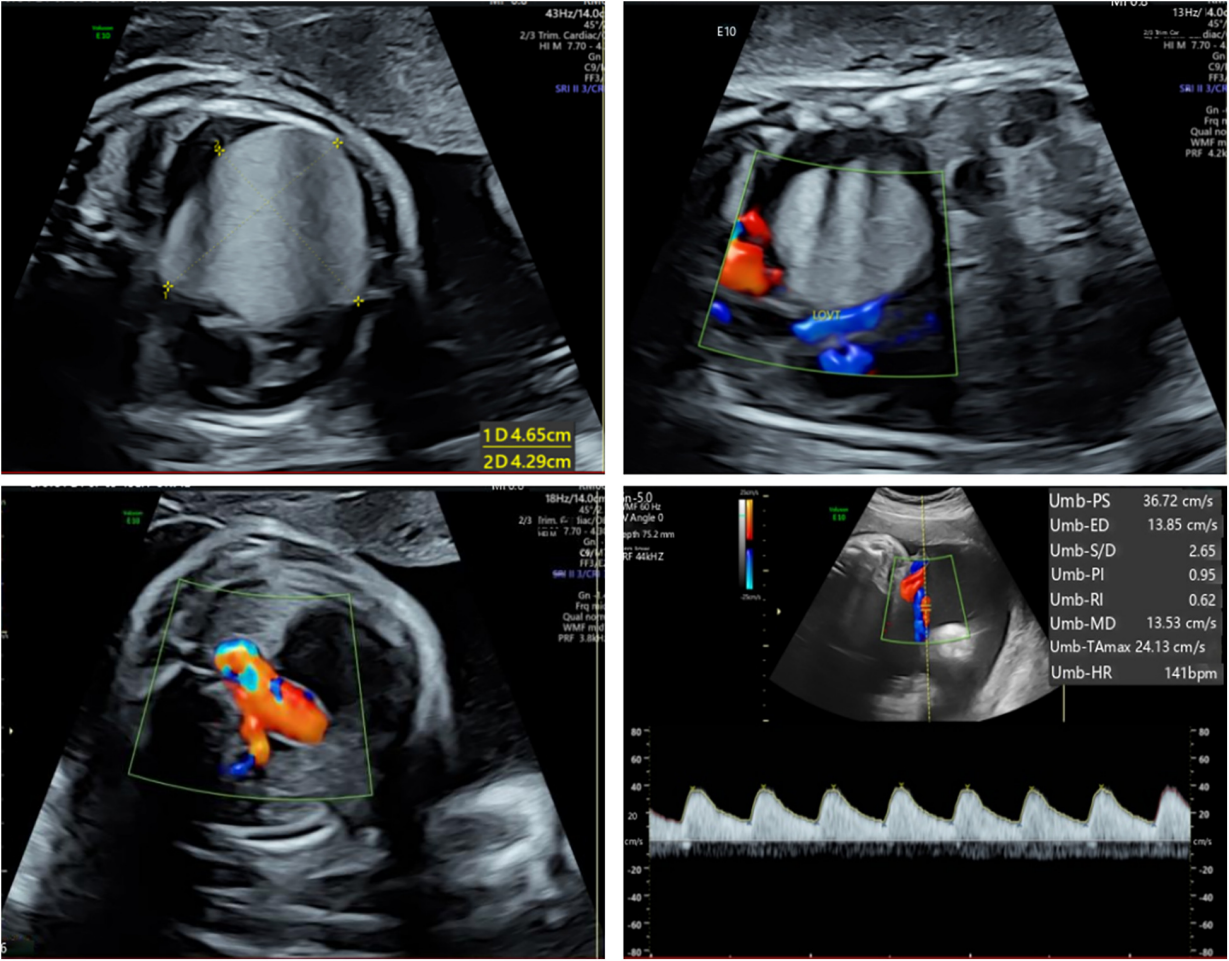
Fetal echocardiography at 34 weeks gestation. Fetal echocardiography detected a hyperechoic mass in the left ventricle, measuring approximately 47 × 44 × 40 mm.
